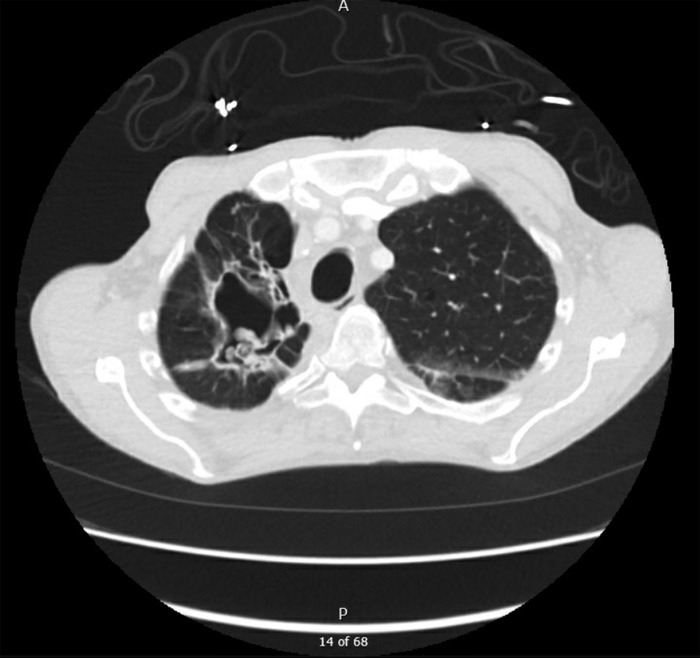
Admission chest computed tomography, transverse view of upper lobes, shows dominant right upper lobe intracavitary lesion. The central component is 2.1 × 2.3 cm (previously 2.0 × 2.3 cm). The mural nodule within this cavitation is 2.1 × 1.2 cm (previously 1.5 × 1.0 cm) and thin walled. Imaging shows progression of right upper lobe bullous emphysema and cystic bronchiectatic changes.
